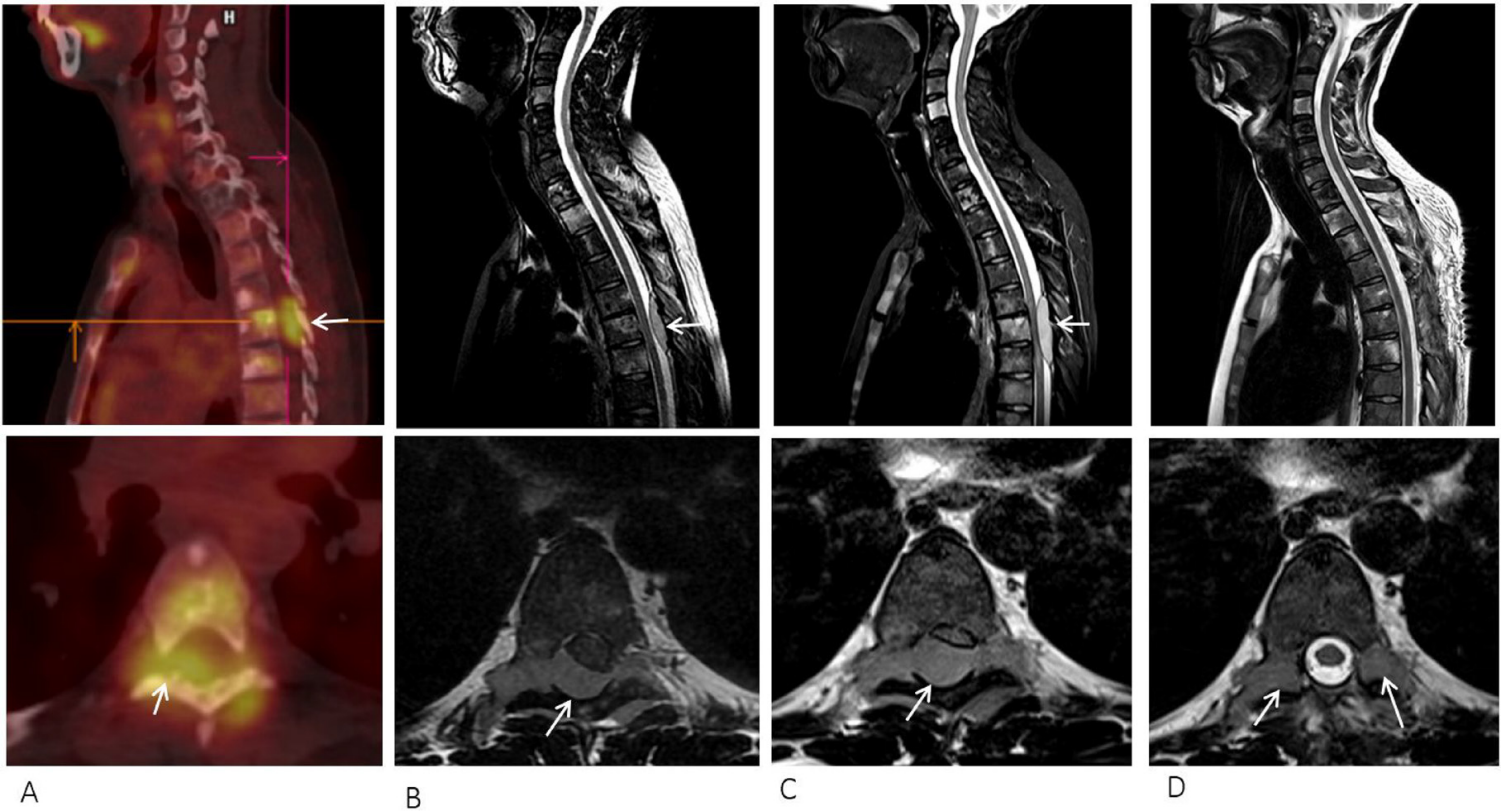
(A) Thoracic epidural metastasis (arrow) detected on PET/CT at progression under temozolomide. T2-weighted MRI scan of the spine at (B) baseline, (C) ten days after starting sonidegib showing tumor progression, and (D) after surgical decompression (raw sagittal and axial images on top and bottom, respectively).
